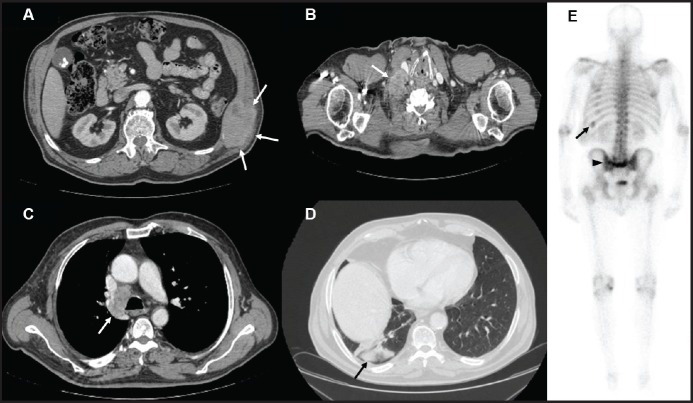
Contrast enhanced CT scan of case presentation. (A) osteolytic mass of the eleventh left rib (white arrows) invading surrounding soft tissues; (B) bulky lymph-nodal metastasis (white arrow) of the right posterior cervical space dislocating the right common carotid artery; (C) lower paratracheal, 4R according to IASLC, lymph-nodal metastasis (white arrow); (D) subpleural mass in the posterior segment of inferior right lobe; (E) Bone scintigraphy showing active pathological uptake of the left eleventh rib (black arrow) and of S2 vertebral body (black arrow head).
